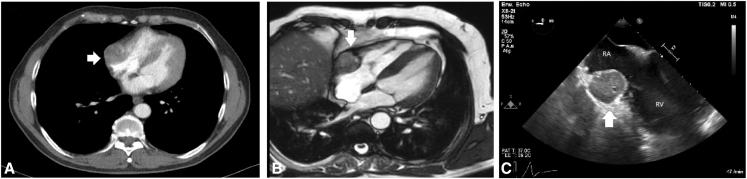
Preoperative chest computed tomography scan (A) and magnetic resonance imaging (B) and intraoperative transesophageal echocardiography (C) demonstrating a 3.0 × 3.7 × 4.0 cm mass involving the right atrium (RA), ventricle (RV), and lateral tricuspid annulus. The *white arrow* shows the tumor.
